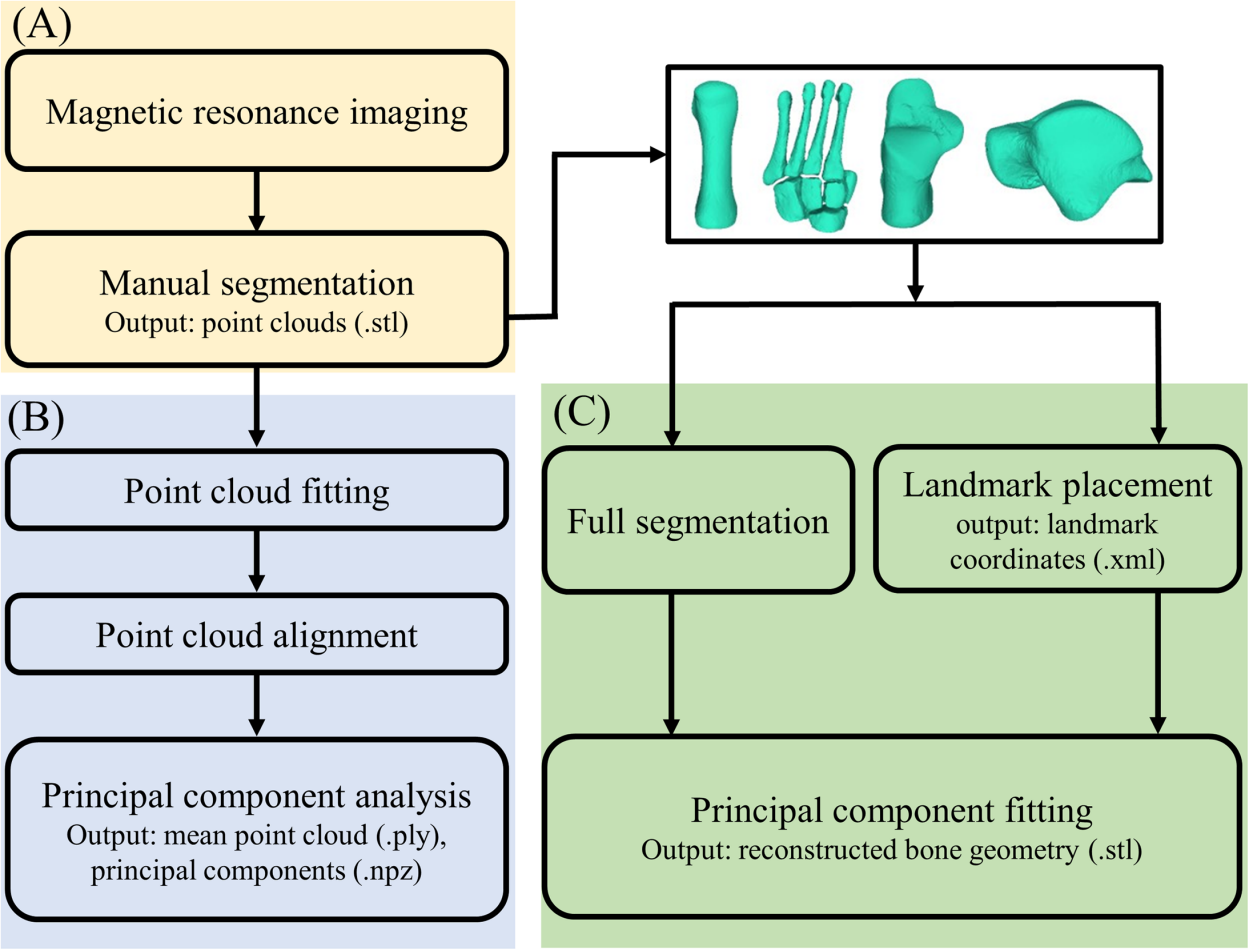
Outline of the process of creating and validating a statistical shape model. (A) Magnetic resonance image processing; (B) statistical shape model generation; and (C) statistical shape model validation.
